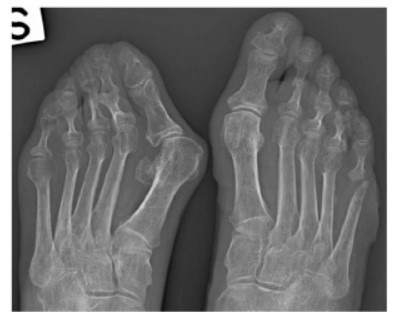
Follow-up radiograph three months later, showing clear signs of osteolysis in the fifth toe of the right foot. Taken on March, 2024.
